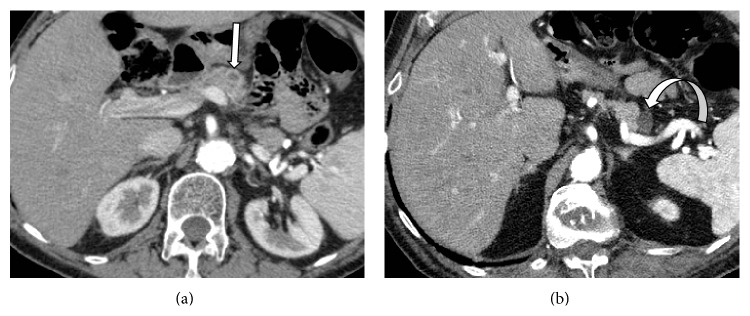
Two examples of pancreatic enhancement and cystic lesions' evidence in abdominal CT angiographic studies. (a) Poor contrast between the pancreatic parenchyma and cyst evident along the anterior edge of the gland (white arrow) and (78-year-old man) arterial phase does not allow proper lesion's evaluation (uncertain wall thickening). (b) Good contrast between pancreatic parenchyma and simple cyst (curved arrow), which demonstrates clear margins (66-year-old man).
